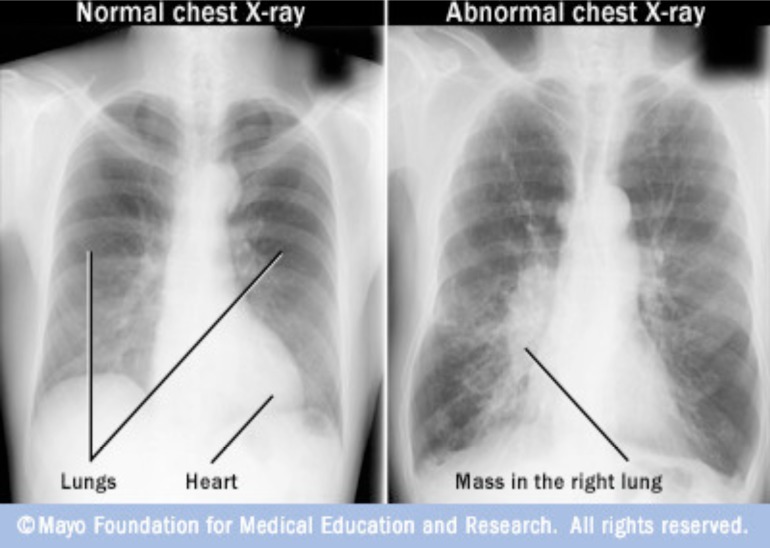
Chest X-Ray of normal lungs and cancer affected lungs [7].
